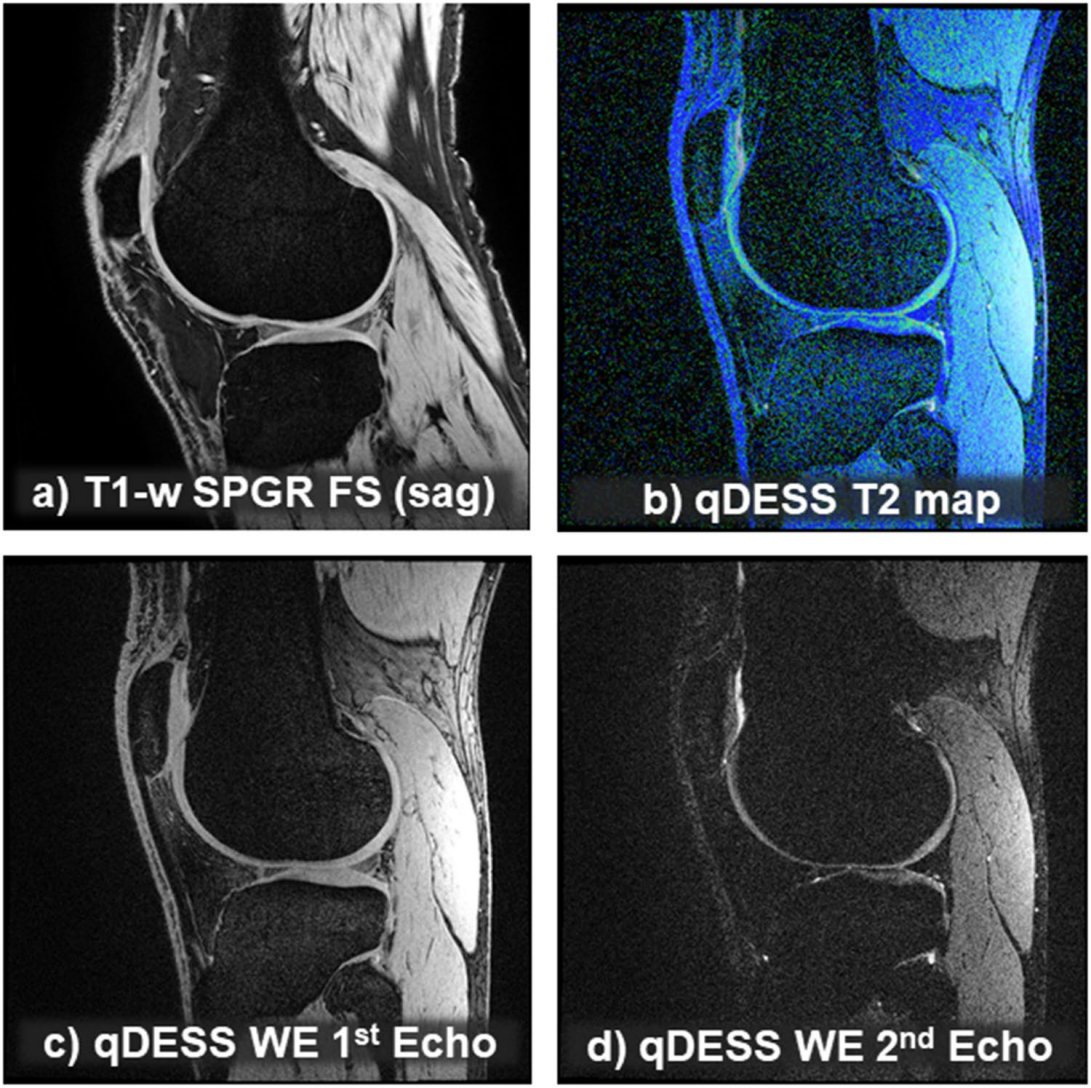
Multifaceted MRI to simultaneously obtain quantitative cartilage morphology and relaxometry (transverse relaxation time [T2]), also suitable for bone size and shape analysis, for meniscus morphometry (if reconstructed in the coronal plane) and potentially for analysis of Hoffa synovitis (under investigation). a Sagittal T1-weighted spoiled gradient echo sequence with fat suppression. b T2 map derived from quantitative dual echo at steady state sequence with water excitation (qDESS) with water excitation (WE), as depicted in b and c (two echos). c qDESS WE (1st echo). d qDESS WE (2nd echo)
